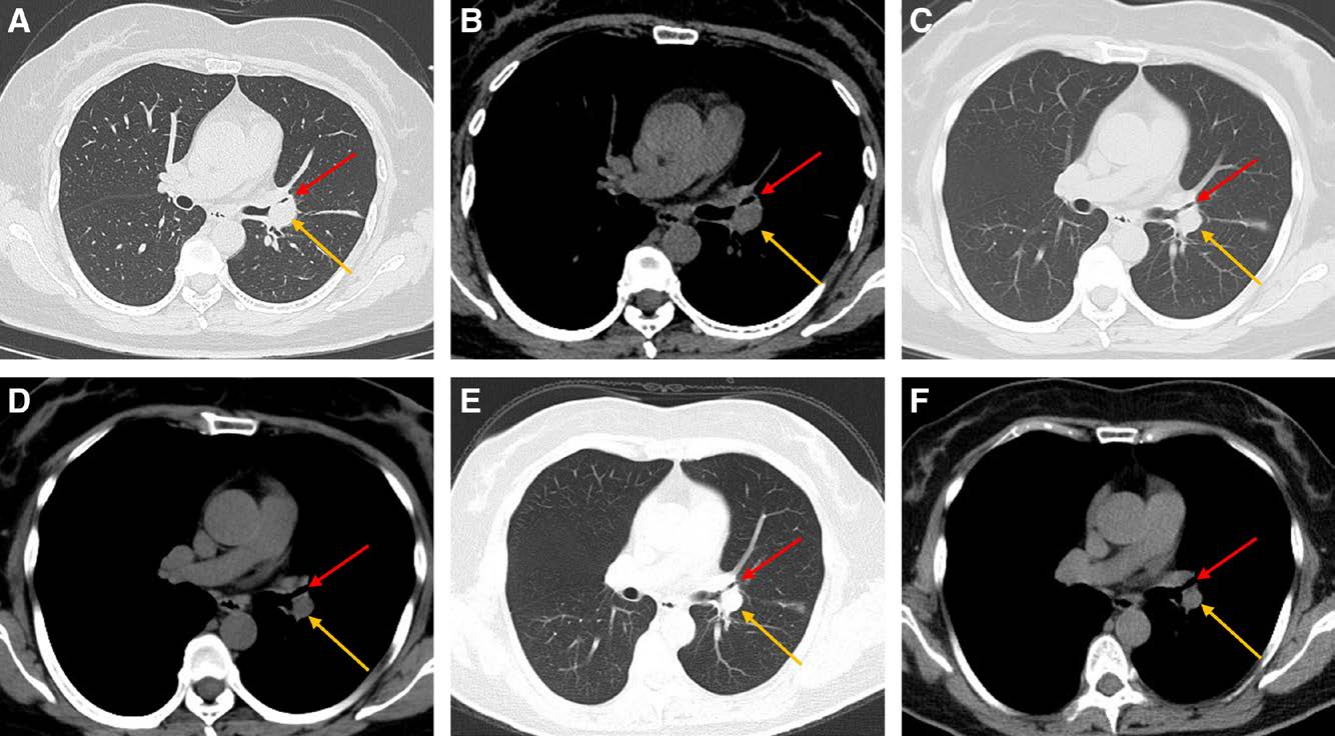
Chest CT findings after treatment with TMP-SMX in patient. (A, B) June 1, 2023, chest CT showed a significant reduction in lesion extent (yellow arrows), and bronchial lumen patency has slightly improved (red arrows). (C, D) Chest CT scan on November 2, 2023. (E, F) Chest CT scan on April 14, 2024. CT = computed tomography, TMP-SMX = trimethoprim-sulfamethoxazole.
